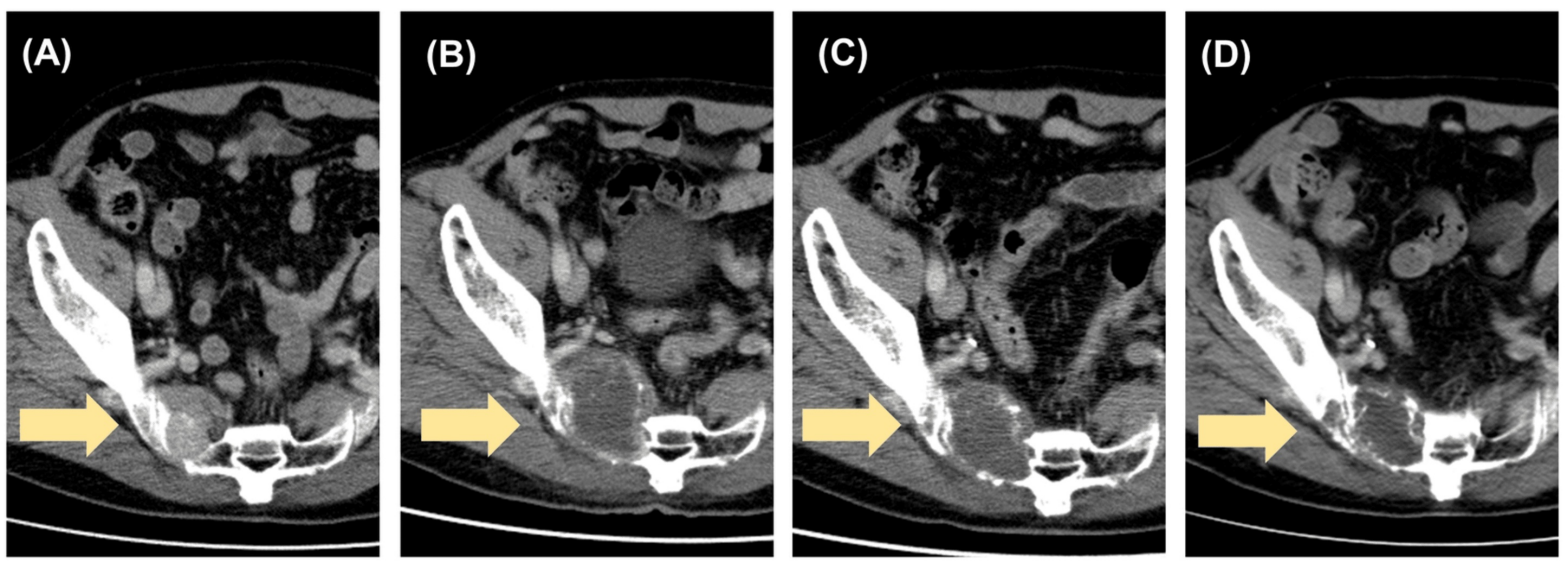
Case of a 74-year-old man with sacral bone metastasis from thymoma The yellow arrows indicated the sacral bone metastasis from thymoma. (A) A computed tomography scan showing a 3-cm mass in the sacral bone at the time of recurrence. (B) After three years of recurrence, the sacral lesion grew to approximately 6 cm. (C) Following stereotactic ablative radiotherapy (SABR), the sacral lesion reduced in size after one year, allowing for a decrease in pain medication. (D) Two years post-SABR, the sacral lesion further reduced in size, and the patient no longer required medication for sacral pain management.
